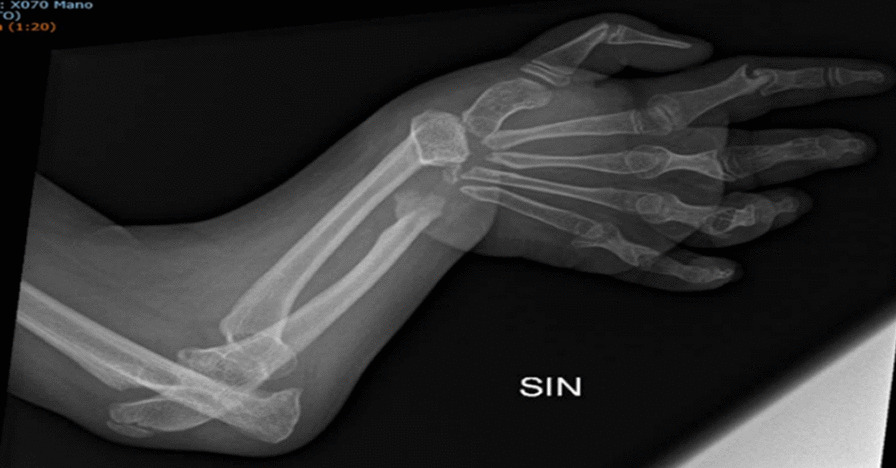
Pt. 2 X-ray at age 14y 5m. Elbow and wrist radiograph showing chronic dislocation with marked dysmorphism of the bony heads, short radius and absence of the carpal bones
